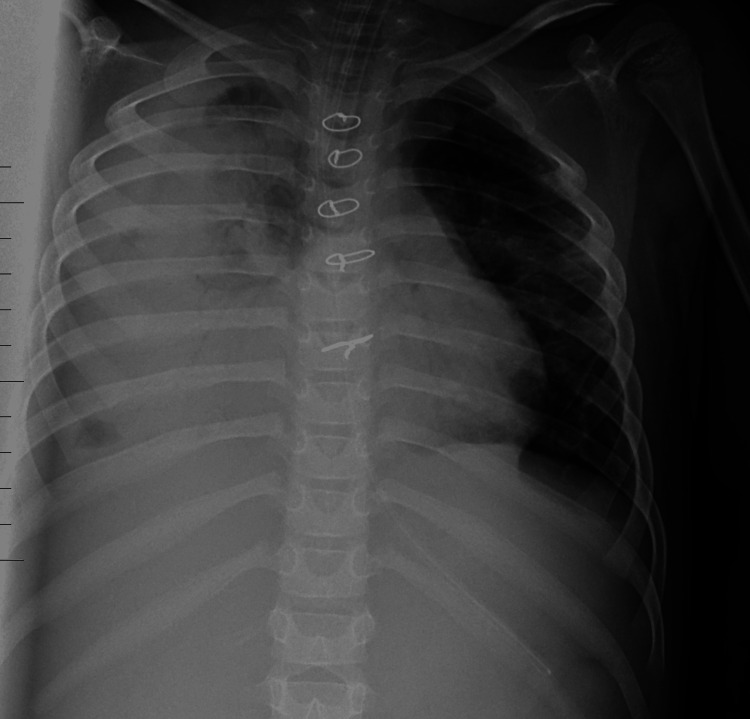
A frontal chest radiograph shows near complete opacification of the right hemithorax with the presence of an air bronchogram, consistent with extensive pneumonia. Sternotomy wires from previous cardiac surgery are seen.
